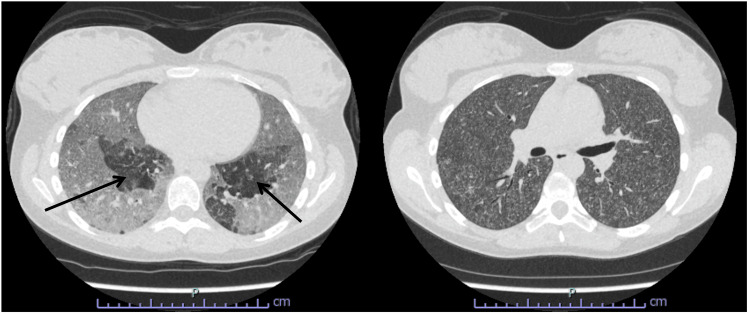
High-resolution CT of the thorax. Diffusely abnormal lungs bilaterally, with innumerable soft tissue centrilobular nodules of varying sizes, most of which are fluffy and ill-defined. In the lower lobes, there are areas of air trapping (see arrows). No mediastinal or hilar adenopathy CT: computed tomography
